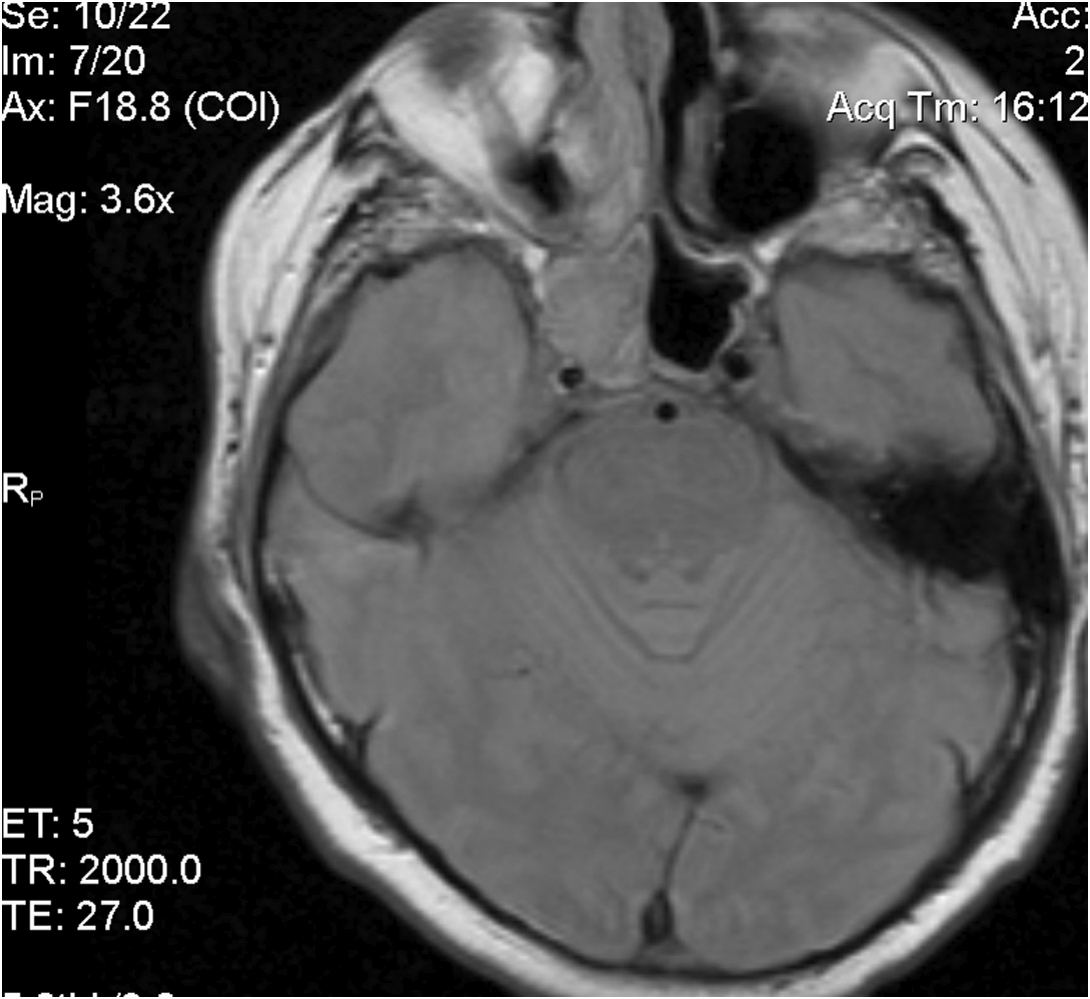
MRI image of mass in sphenoid sinus/optic nerve involvement transverse
